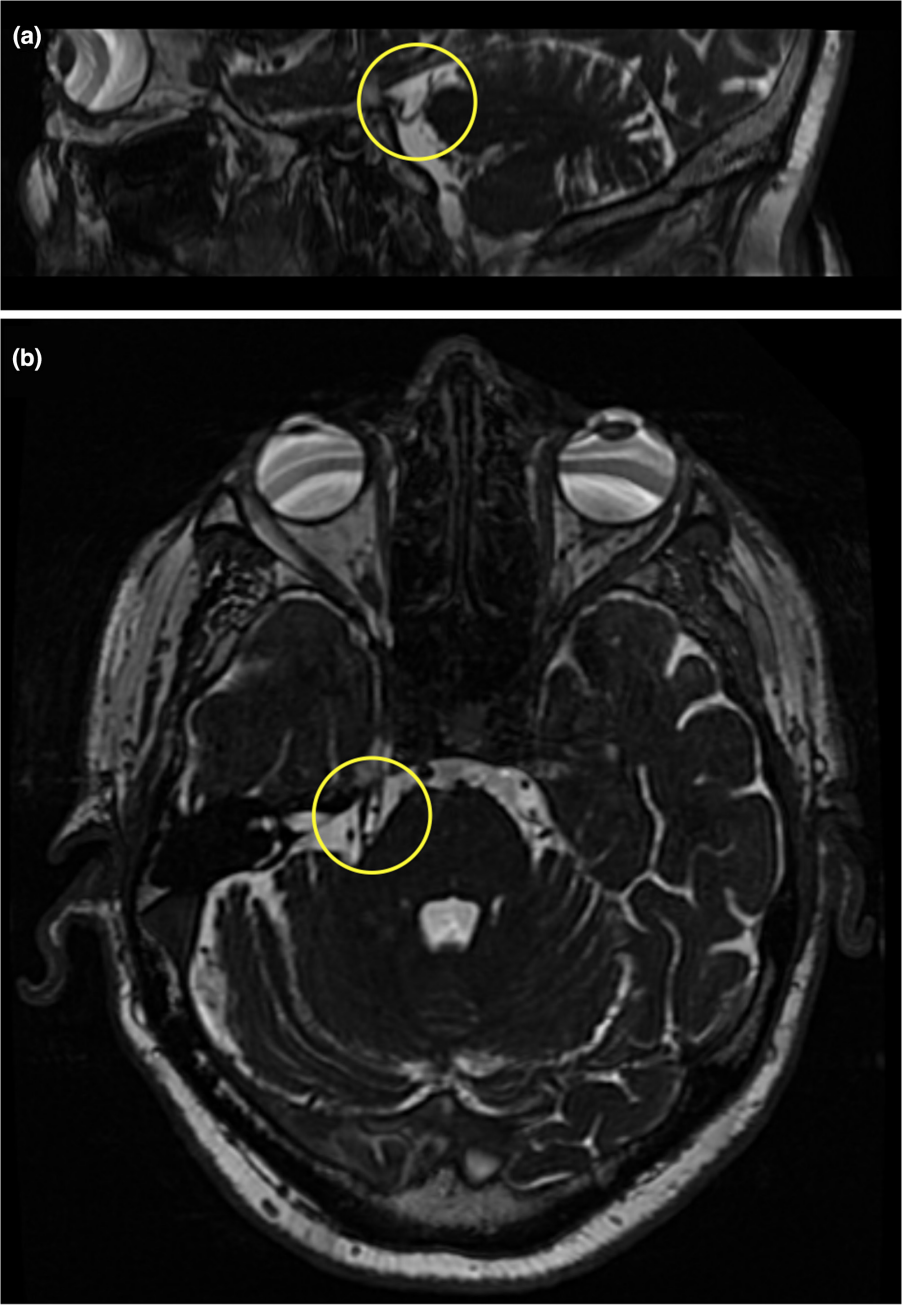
Sagittal (a) and axial (b) T2-weighted magnetic resonance images demonstrating neurovascular conflict on the right side (yellow circle) between the trigeminal nerve and a descending loop of the superior cerebellar artery.
